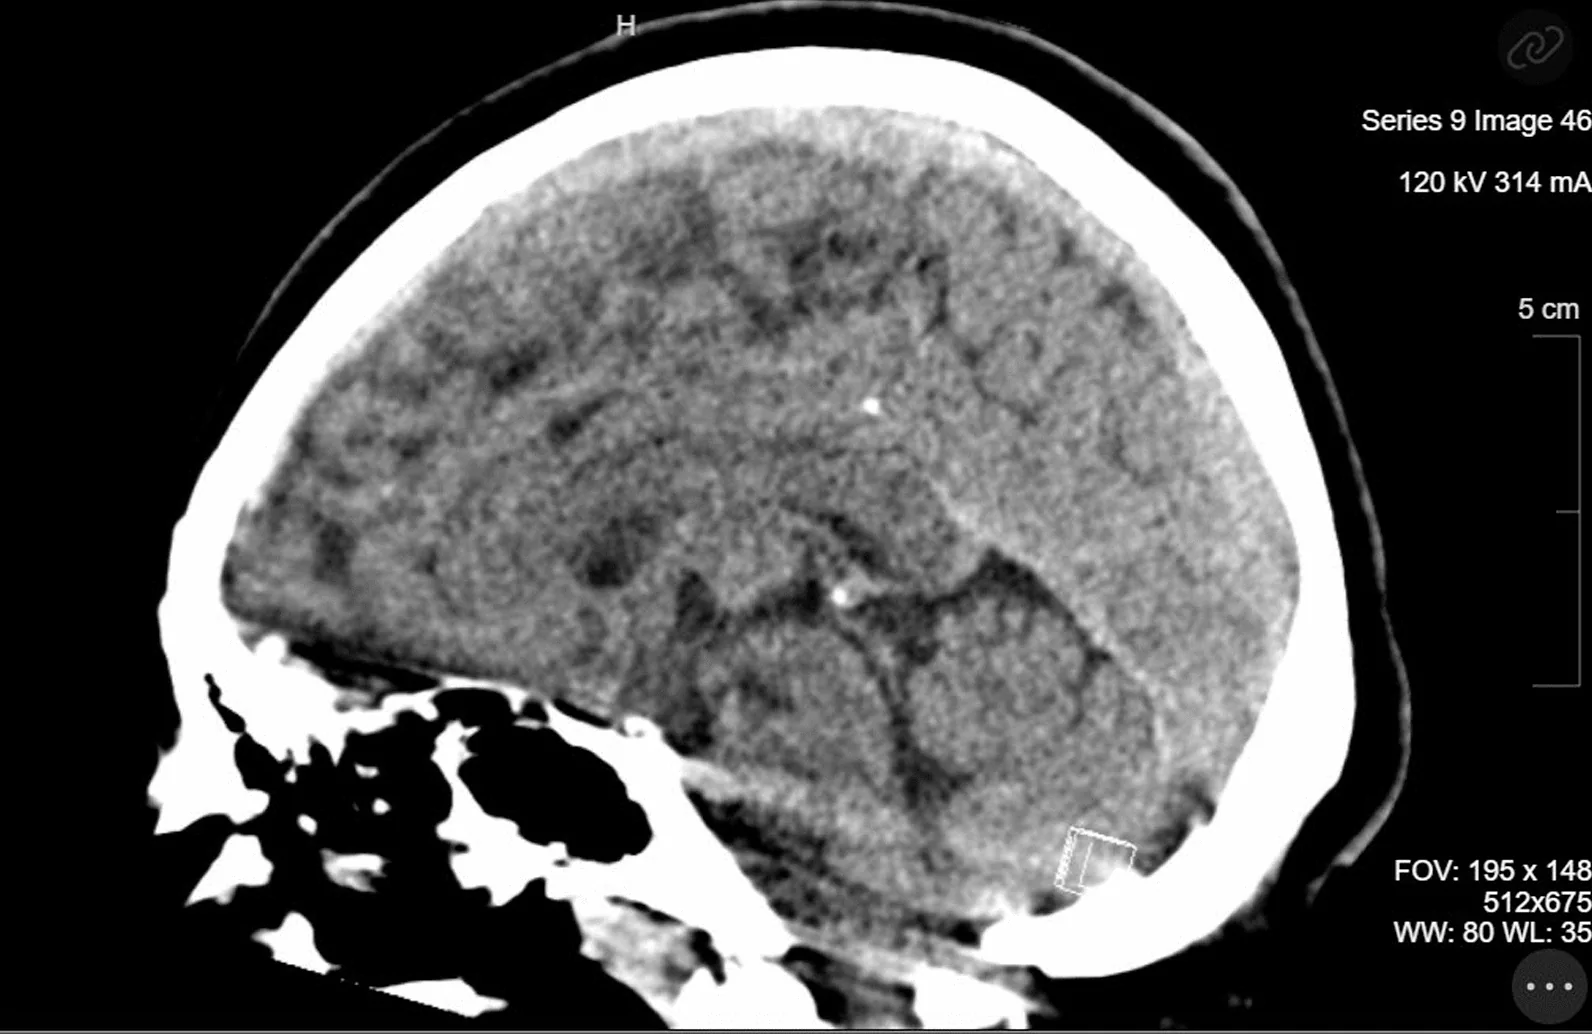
Sagittal CT brain (non-contrast) demonstrating no evidence of pituitary adenoma, mass lesion, or optic chiasmal compression
